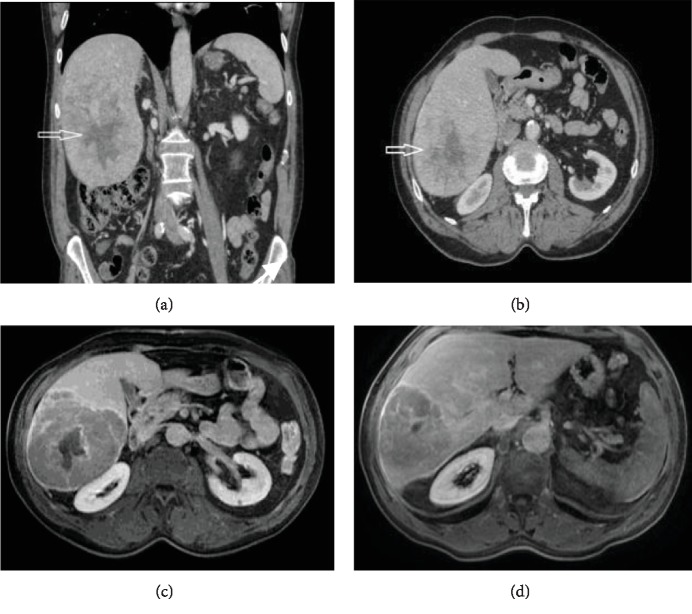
Coronal (a) and axial (b) CT scan images showing a large (>10 cm) inhomogeneously enhancing liver mass with a central scar occupying liver segments V, VI, and VII (arrow). (c, d) Axial post contrast MRI image demonstrating heterogeneously enhancing liver mass with washout and central nonenhancing component, features mostly consistent with atypical HCC.
